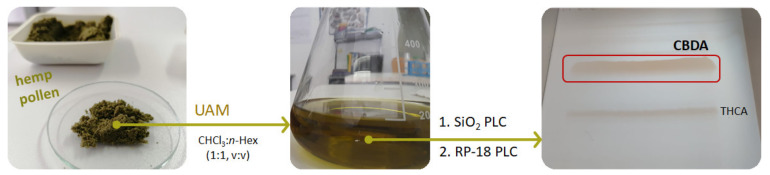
Hemp pollen underwent ultrasound accelerated maceration (UAM), and an aliquot of hemp pollen (HP) extract (60 mg) was then chromatographed by preparative thin-layer chromatography (PLC) using a precoated silica gel 60 F254 (20 × 20 cm, 1 mm, Merck, Darmstadt, Germany). The organic lower phase of a biphasic CHCl_3_/MeOH/H_2_O (13:7:7, *v/v/v*) solution served as mobile phase. Among the five fractions obtained, one was further fractionated by thin-layer chromatography using a precoated silica gel 60 RP-18 F254S (20 × 20 cm, Merck, Darmstadt, Germany). Elution was twice by MeCN/H_2_O (4:1, *v/v*) solution. The main compound (yield 45%) was identified, by means of spectroscopic and spectrometric techniques, as cannabidiolic acid, whereas the other compound was THCA-A.
